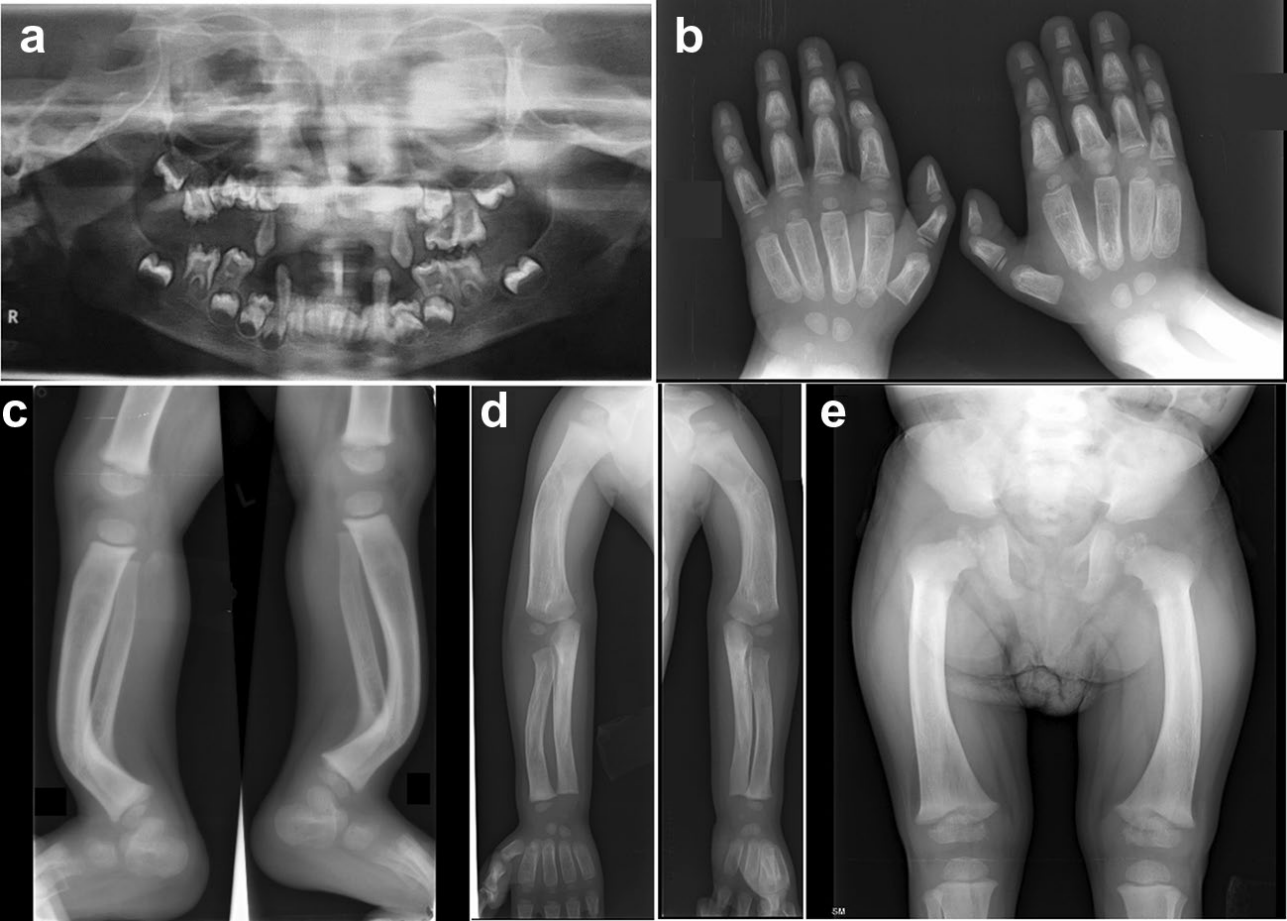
Radiographic profile of an individual with vitamin-D-dependent rickets type I. P1 (age: 4 y) shows delayed tooth eruption at 8 y 9 mo (a), delayed carpal ossification (b), metaphyseal dysplasia at the ends of long bones (c–e): small epiphyses at the knee (c), bending of long bones (c, d), and small capital femoral epiphysis (e). The radiographic appearance was affected by his treatment
